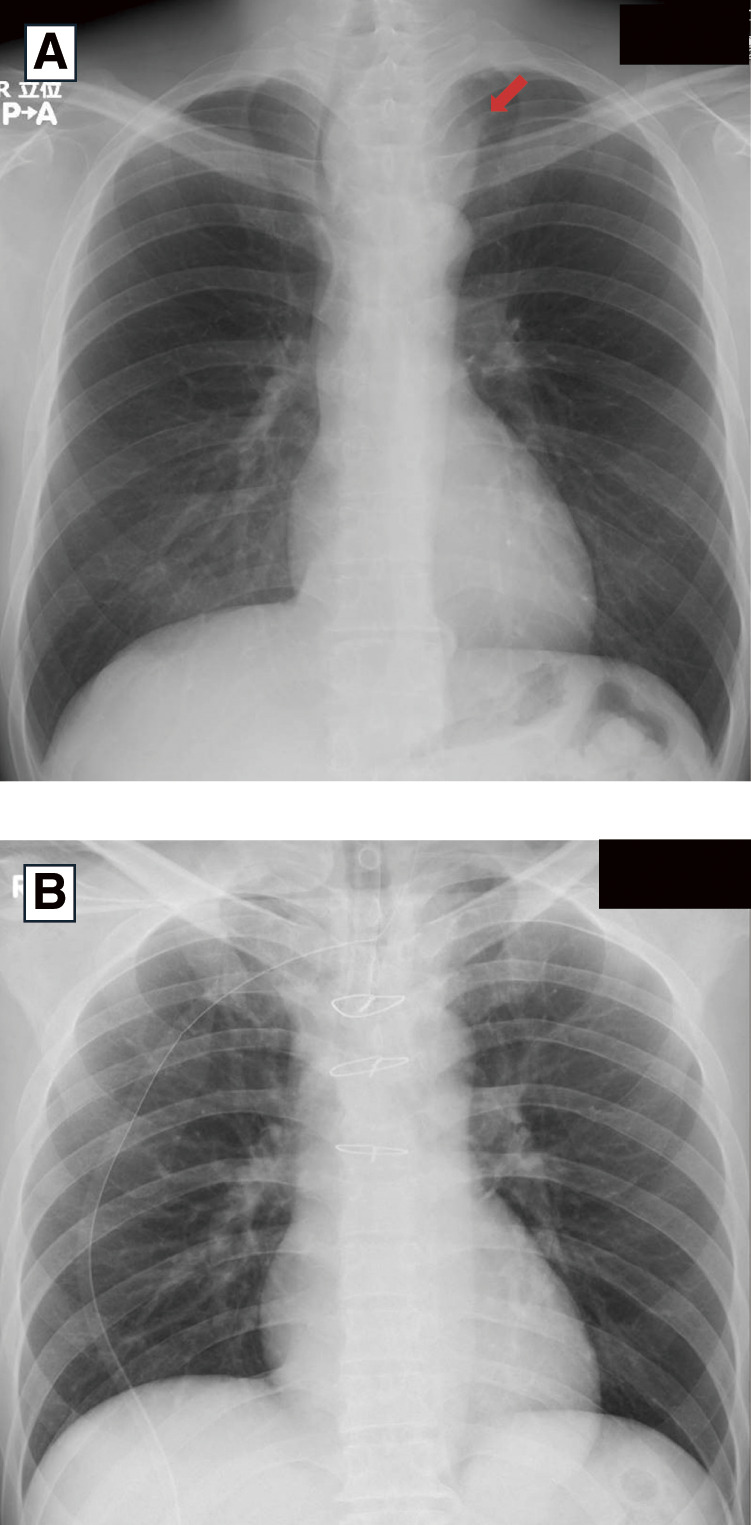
Chest radiography findings. (A) Chest radiography reveals a mass (arrow) on the left side of the trachea, which causes tracheal deviation to the right side of the trachea. (B) Postoperative chest radiograph showing improvement in tracheal deviation.
